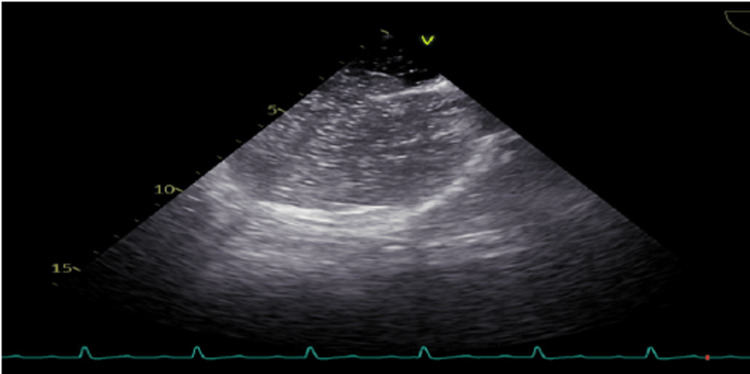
Transesophageal echocardiogram (TEE) demonstrating right-to-left shunt through patent foramen ovale on TEE with bubble study
